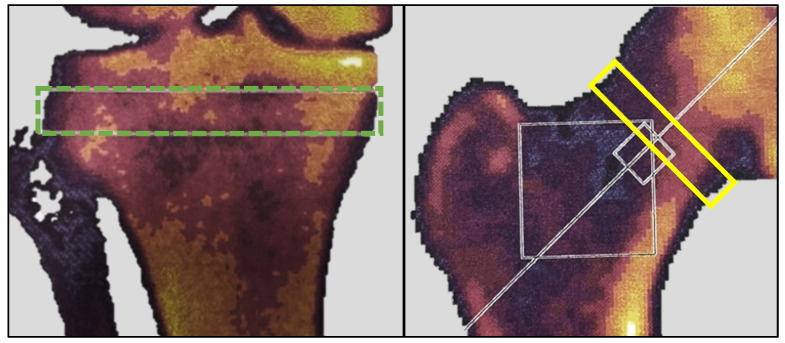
DEXA measurements. The green dotted box represents the area of BMC and BMD measurements. The yellow box represents the area of T-score measurement. BMC, bone mineral content; BMD, bone mineral density; DEXA, dual-energy X-ray absorptiometry.
